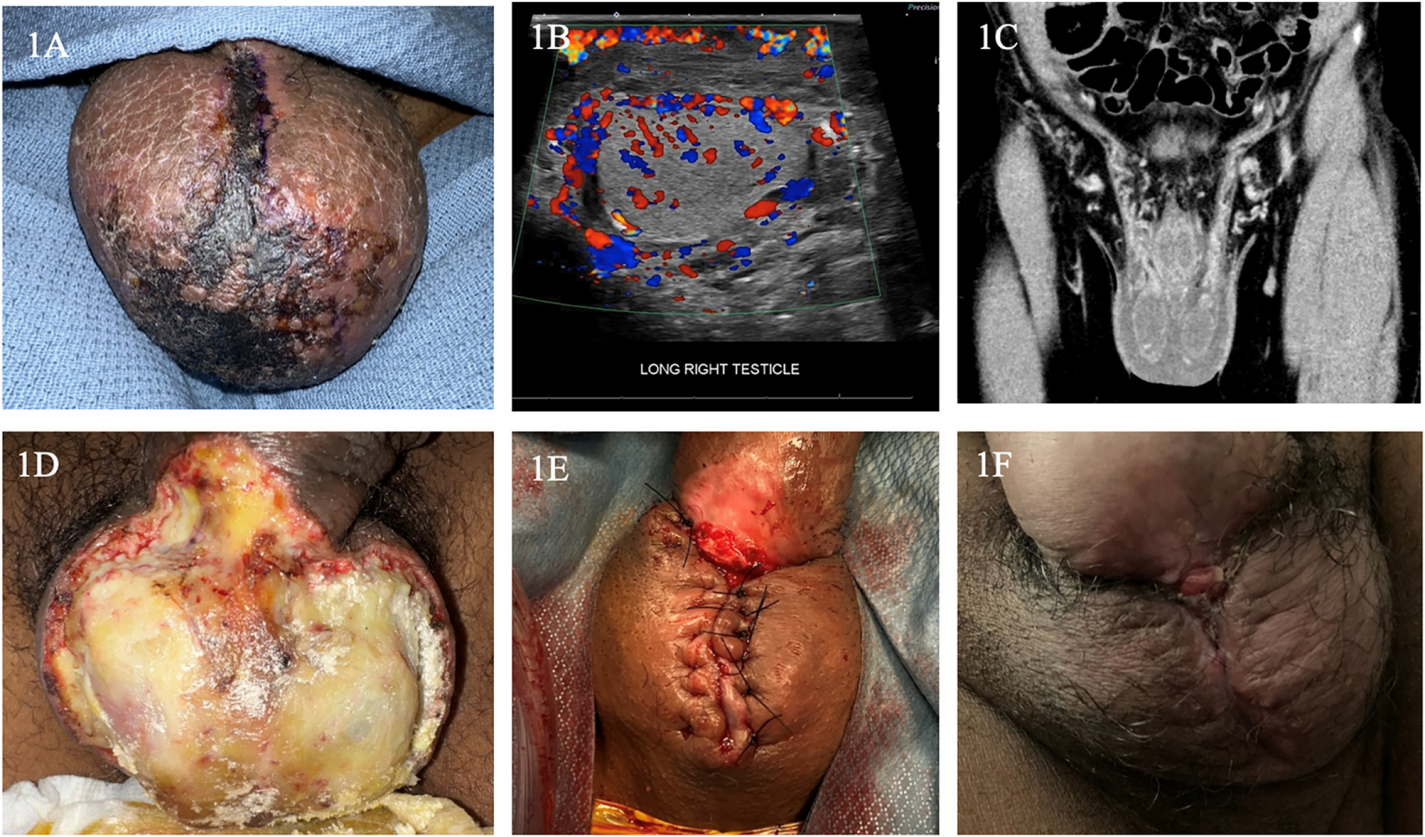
Pictorial timeline: Mitomycin C-induced full thickness genital cutaneous necrosis, diagnosis and treatment. (A) Genital cutaneous necrosis that initially developed along median raphe to fulminant bilateral scrotal involvement and eschar formation as viewed anteriorly on POD 75. (B) Ultrasound image on initial presentation with scrotal pain demonstrating thickened, hyperemic scrotal skin and lymphedema with normal testes. (C) Coronal image from CT with IV contrast demonstrates retroperitoneal infiltration along the testicular veins (red arrowheads), one hypothesized pathogenesis of genital necrosis. (D) Scrotal wound after multiple debridement’s of full thickness genital cutaneous necrosis on POD 81 following complete demarcation. Notable underlying tunica albuginea and testicles intact without evidence of further necrosis or infection. (E) Scrotal wound status post primary closure POD 185. (F) Scrotal wound as seen on POD 237, approximately 7 weeks following primary closure, demonstrating excellent healing and cosmesis.
